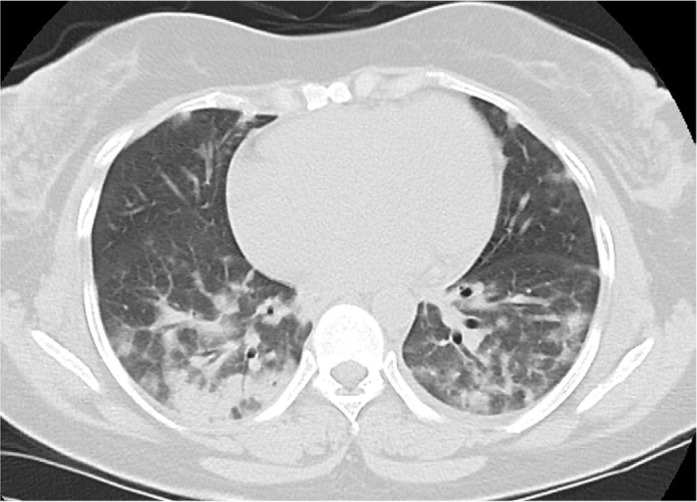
She was diagnosed with antisynthetase syndrome presenting primarily with ILD. The patient was started on intravenous pulse dose steroids (1gm methylpredniso-lone) along with mycophenolate mofetil (MMF) resulting in significant improvement in her clinical symptoms. She was discharged home on tapering steroids, MMF, and oxygen supplementation with close follow up. Outpatient treatment with rituximab allowed her to be weaned off oxygen supplementation and eventually regain her baseline level of activity. A follow up computed tomography of the chest showed interval decrease in her bibasilar infiltrates (Figure 3). Her initial and 5-month post-treatment pulmonary function tests showed an improvement in D_LCO_ and FVC (Table 1).
